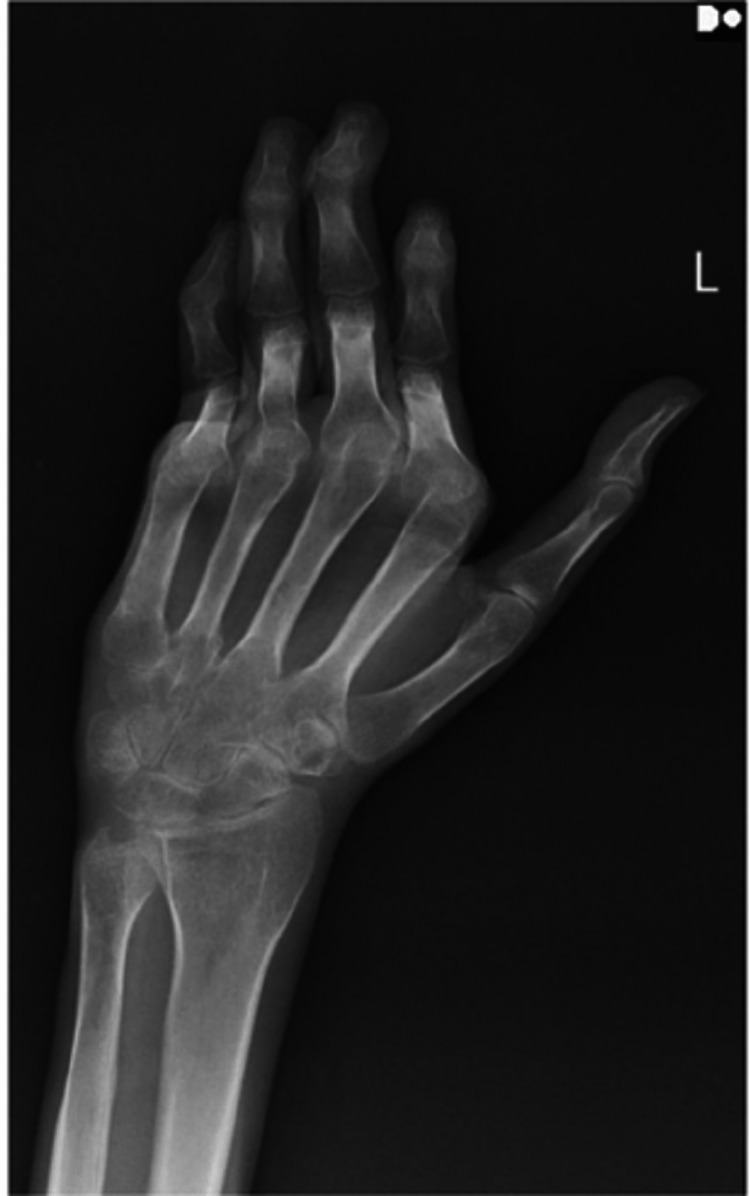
X-ray revealing nonerosive deformity.
